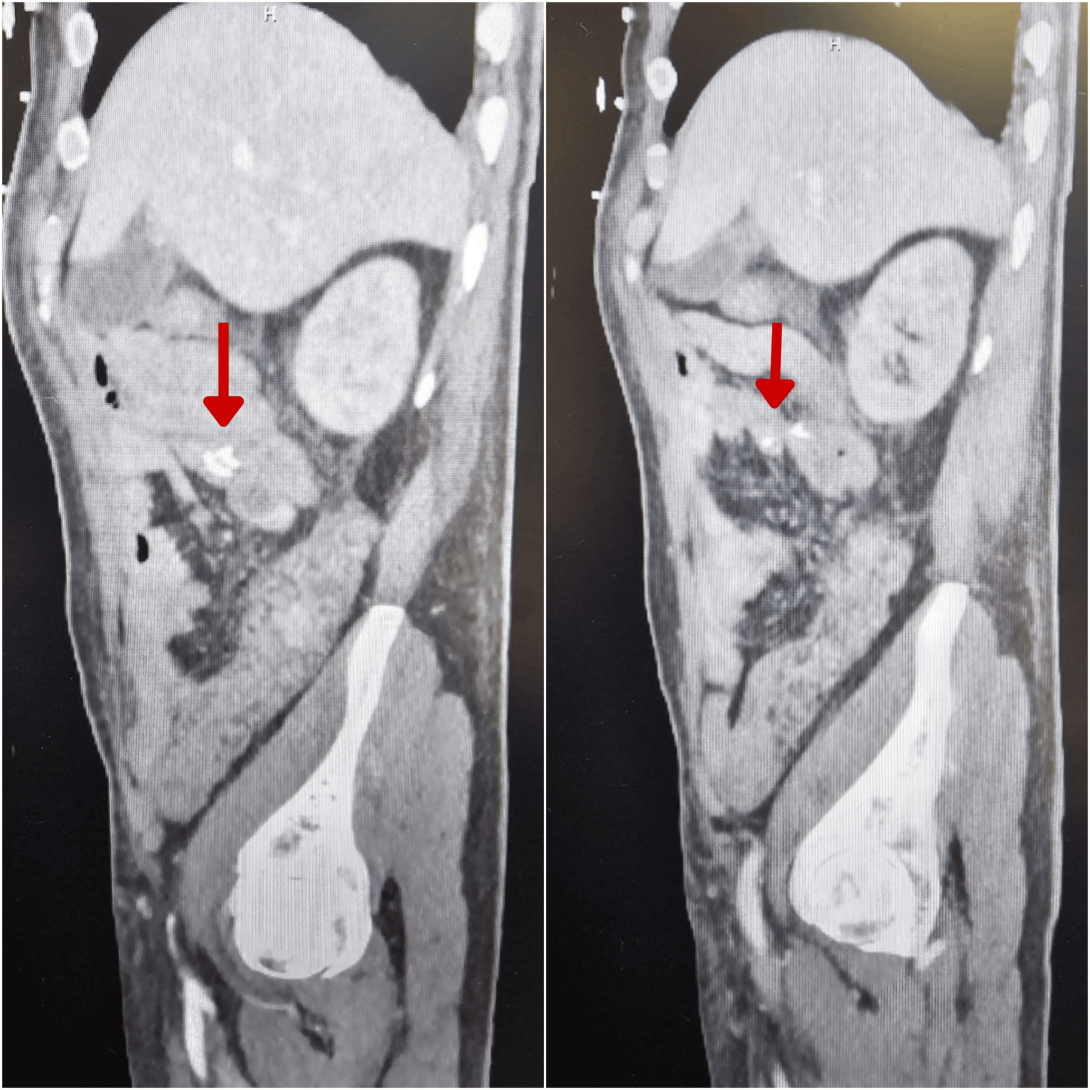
CT angiogram of the abdomen and pelvis showing a blush of intraluminal contrast adjacent to anastomotic structures in the right upper abdomen concerning for GI bleed (arrows).
